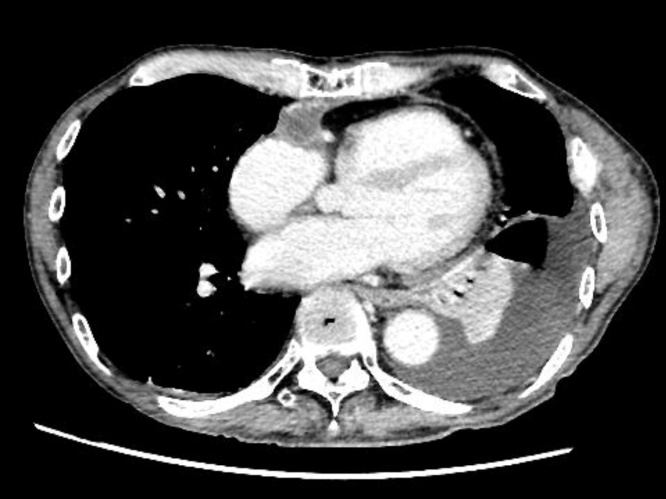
Enhanced computed tomographic scan showed the right atrial wall lumen was not narrowed. Pleural and pericardial effusion are observed.
